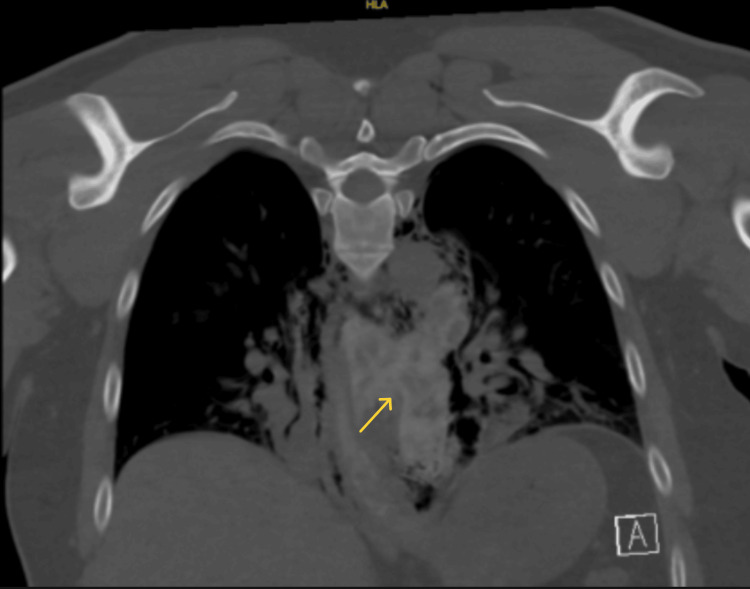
CT chest coronal view showing spillage of esophageal contents to the mediastinum
